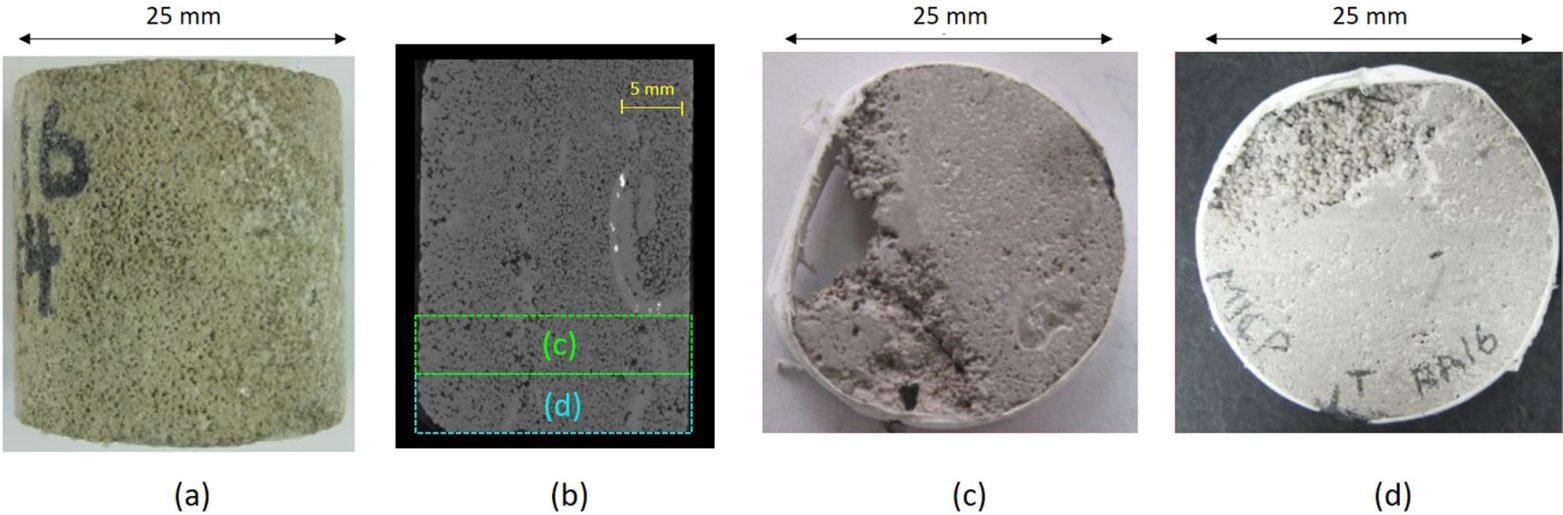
HPMI experiments subsample selection and image acquisition at high resolution for Sample S_1_: (a) core plug photograph, (b) X-Ray 3D-MCT at 20 μm resolution and selection by visual inspection of subsamples, (c) Subsample (trim) to be examined at high resolution, and (d) Subsample used for HPMI measurements.
